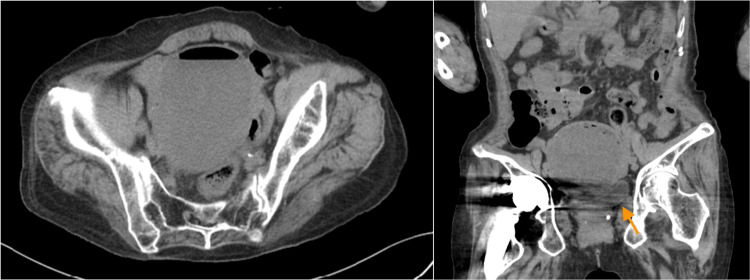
Non-contrast computed tomography of the abdomen. A 10 cm large cystic lesion with air inside is seen on the pelvic floor. It is compressing the bladder (arrow).
